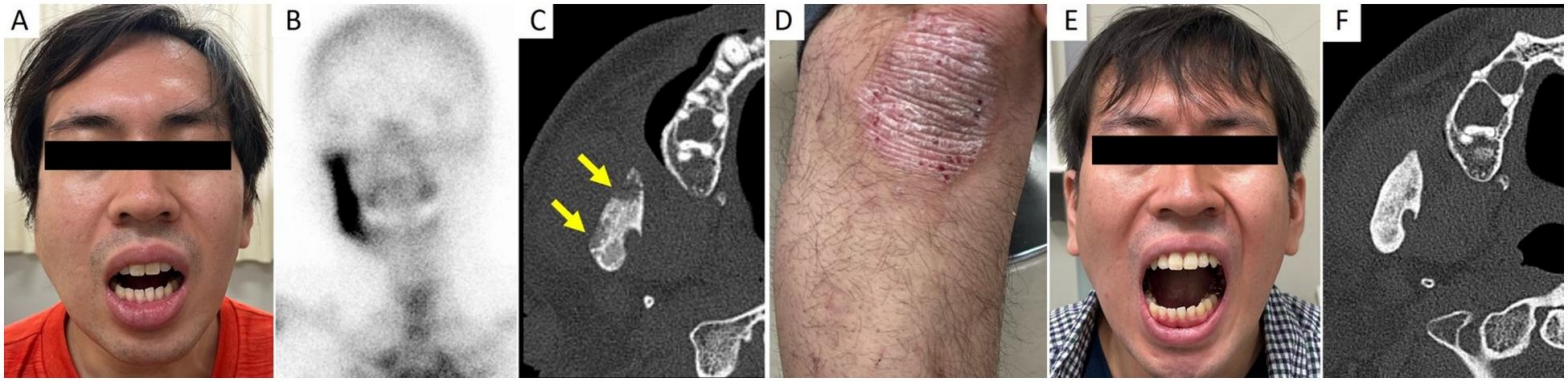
Imaging findings of the patient. (A) Swelling of the right jaw and trismus. (B) Bone scintigraphy findings show increased uptake in the right mandible. (C) CT reveals osteolytic lesions of right mandible (arrow). (D) Psoriatic plaque observed in the right knee. (E) Swelling of the right jaw and trismus improved after administration of certolizumab pegol (4 weeks after initiation of treatment). (F) CT reveals the improvement of osteolytic lesions of right mandible (5 months after initiation of treatment)
